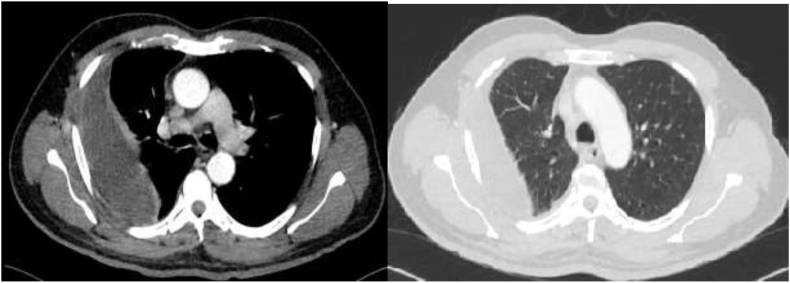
Computed tomography of the chest showing moderate right-sided effusion with normal lung parenchyma.
